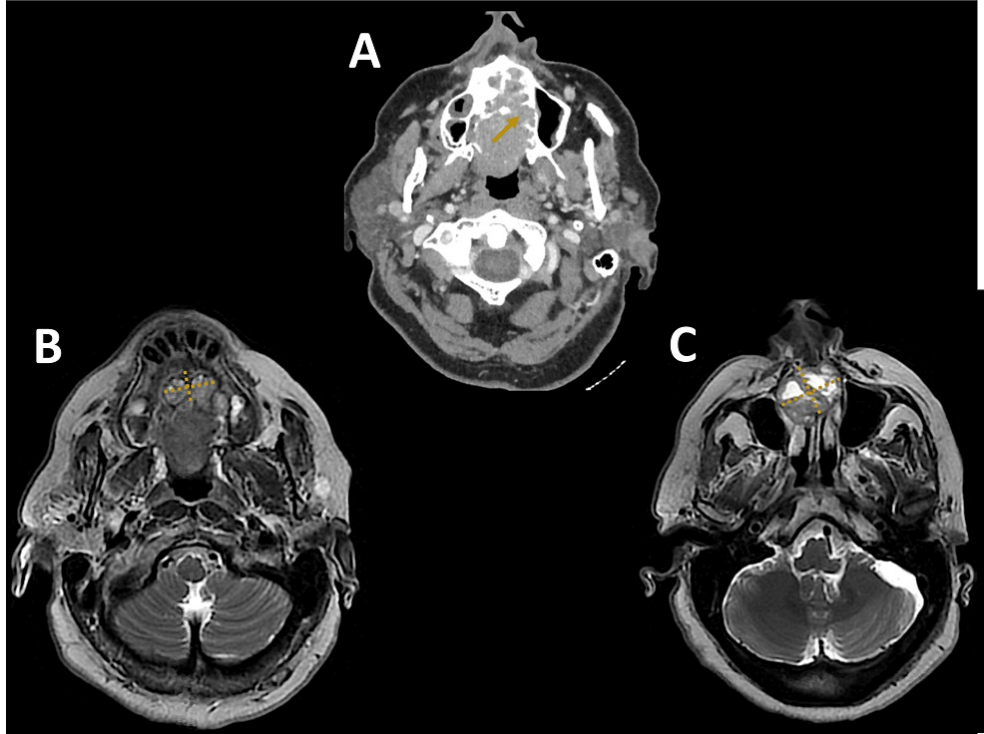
MRI A and B: T1 gadolinium craniofacial MRI shows a tumor taking up gadolinium heterogeneously, centered on the hard palate and extending posteriorly to the soft palate. (The yellow arrow shows bone invasion in the hard palate, and the yellow cross shows the tumor’s limits.) C: T1 gadolinium craniofacial MRI shows the extension of the lesion above and in front of the right and left nasal cavities and involvement of the septum and the lower part of the maxillary sinus. (The yellow cross shows the tumor’s limits). MRI: magnetic resonance imaging
